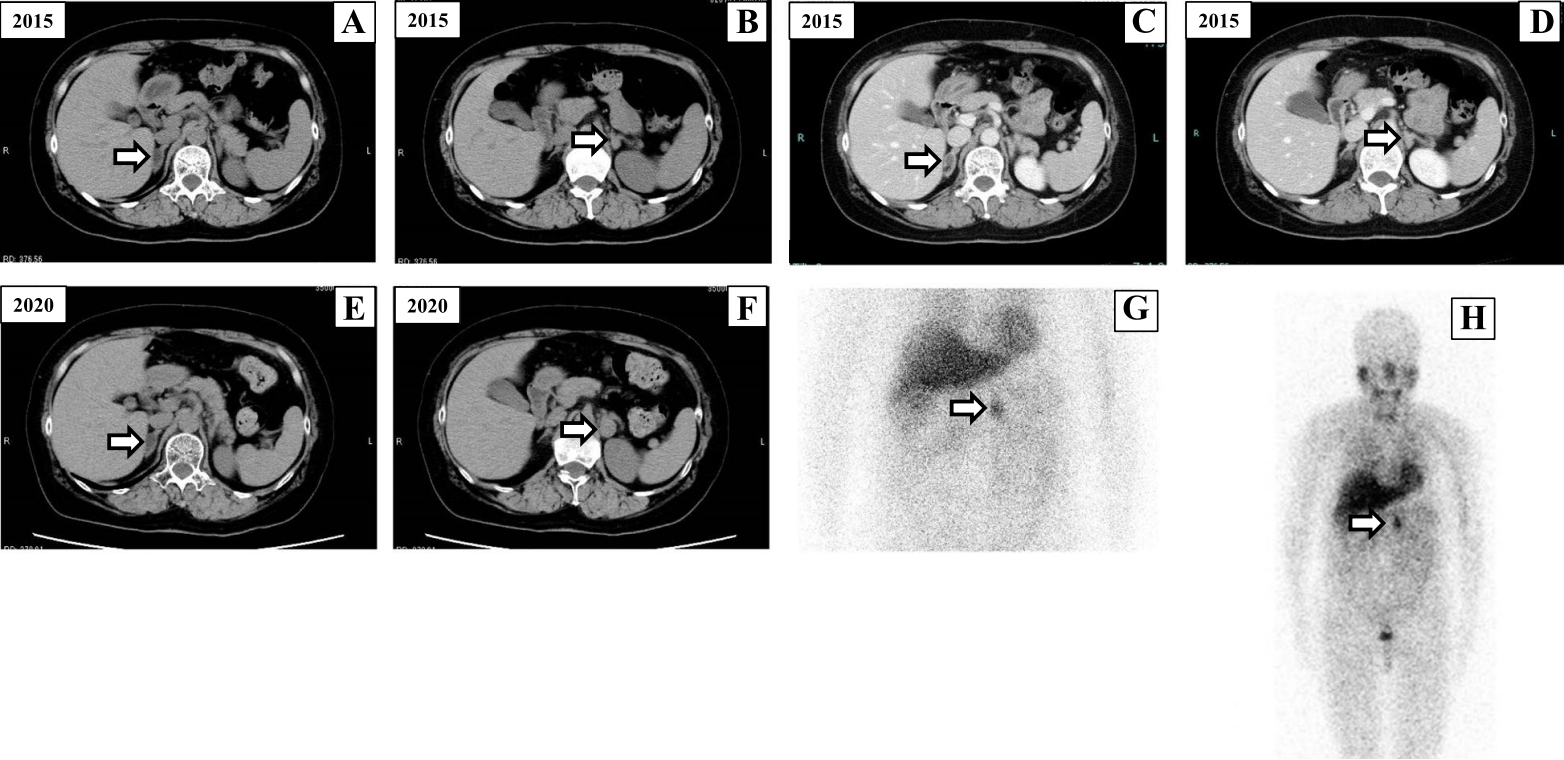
A and B Computed tomography (CT) during the initial adrenal vein sampling (AVS) revealed bilateral adrenal nodules (right:16 × 11 mm, left:13 × 9 mm) with CT attenuation values of 30–60 Hounsfield units on the left and 10 Hounsfield units on the right. C and D Contrast-enhanced CT showed contrast effect in the left adrenal nodule but not in the right. E and F CT images at the second AVS (performed 5 years after the initial AVS) showed no significant changes in the right adrenal mass and enlargement of the left adrenal nodule (26 × 13 mm). G and H
